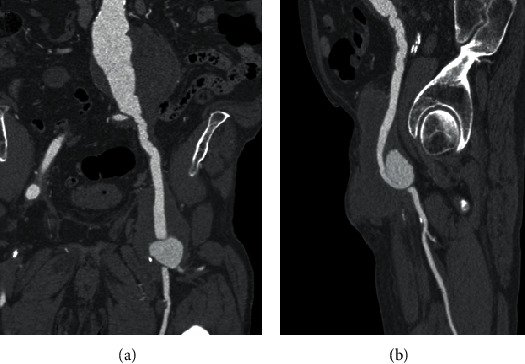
Patient's CT angiography on admission: (a) asymptomatic 11 cm juxtarenal abdominal aortic aneurysm with partially thrombosed 65 × 55 mm pseudoaneurysm in the left groin; (b) partially thrombosed 65 × 55 mm pseudoaneurysm in the left groin.
